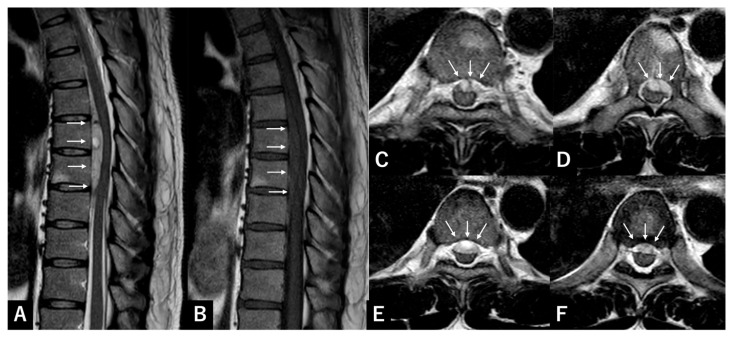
Representative case of SSEH. (A) T2-weighted sagittal MRI of the thoracic spine showing a ventral spinal hematoma (arrow) at T6–T8; (B) T1-weighted sagittal plane; (C) T2-weighted axial plane at T5/6; (D) T6; (E) T6/7; (F) T7/8. SSEH: spontaneous spinal epidural hematoma; MRI: magnetic resonance imaging.
